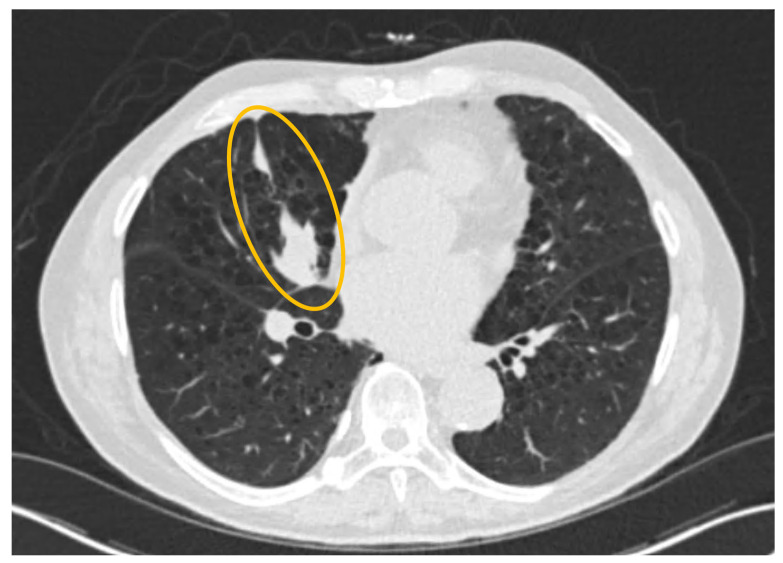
Non-contrast CT chest (lung window): Moderately severe centrilobular emphysema with a yellow circle highlighting the right middle lobe subsegmental atelectasis.
